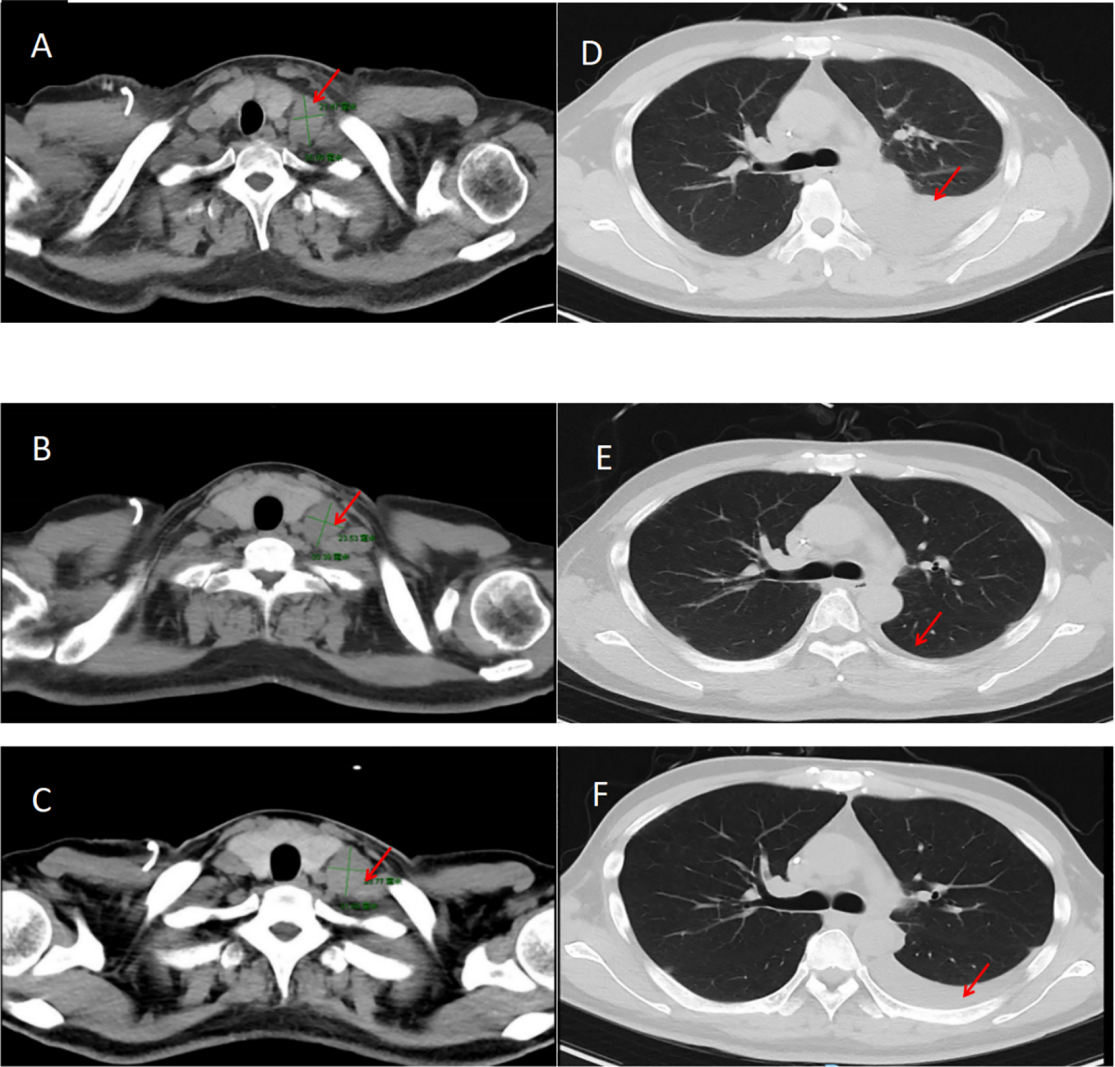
(A) Neck CT in January 2023 showing a supraclavicular lymph node measuring 21.47x35.09 mm. (B) CT of the neck in March 2023 showing the resolution of the supraclavicular lymph node involvement, and a size of ~23.53x30.39 mm. (C) Chest CT in May 2023 showing a supraclavicular lymph node size of ~23.77x31.69 mm. (D) CT of the chest in January 2023 showing a left-sided pleural effusion. (E) CT of the chest in March 2023 showing resolution of the pleural effusion. (F) CT of the chest in May 2023 showing that the pleural effusion is largely gone. CT, computed tomography.
